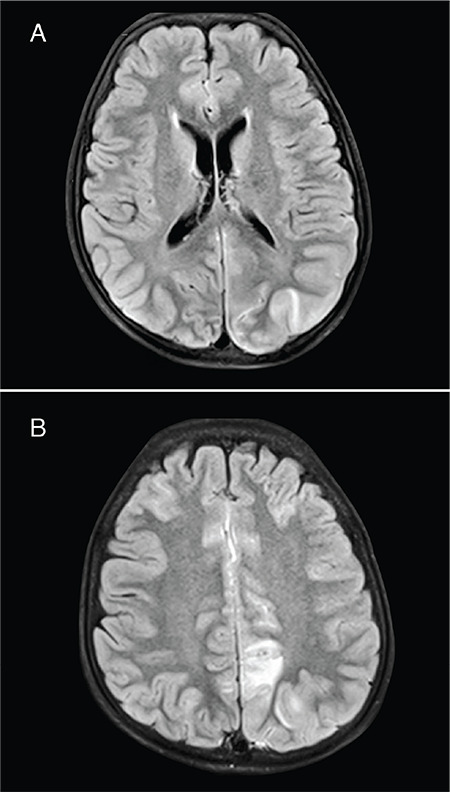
A, B) Magnetic resonance imaging of brain (first case) with T2/fluid-attenuated inversion recovery images showing hyperintensities in the parieto-occipital regions, predominantly on the left side
